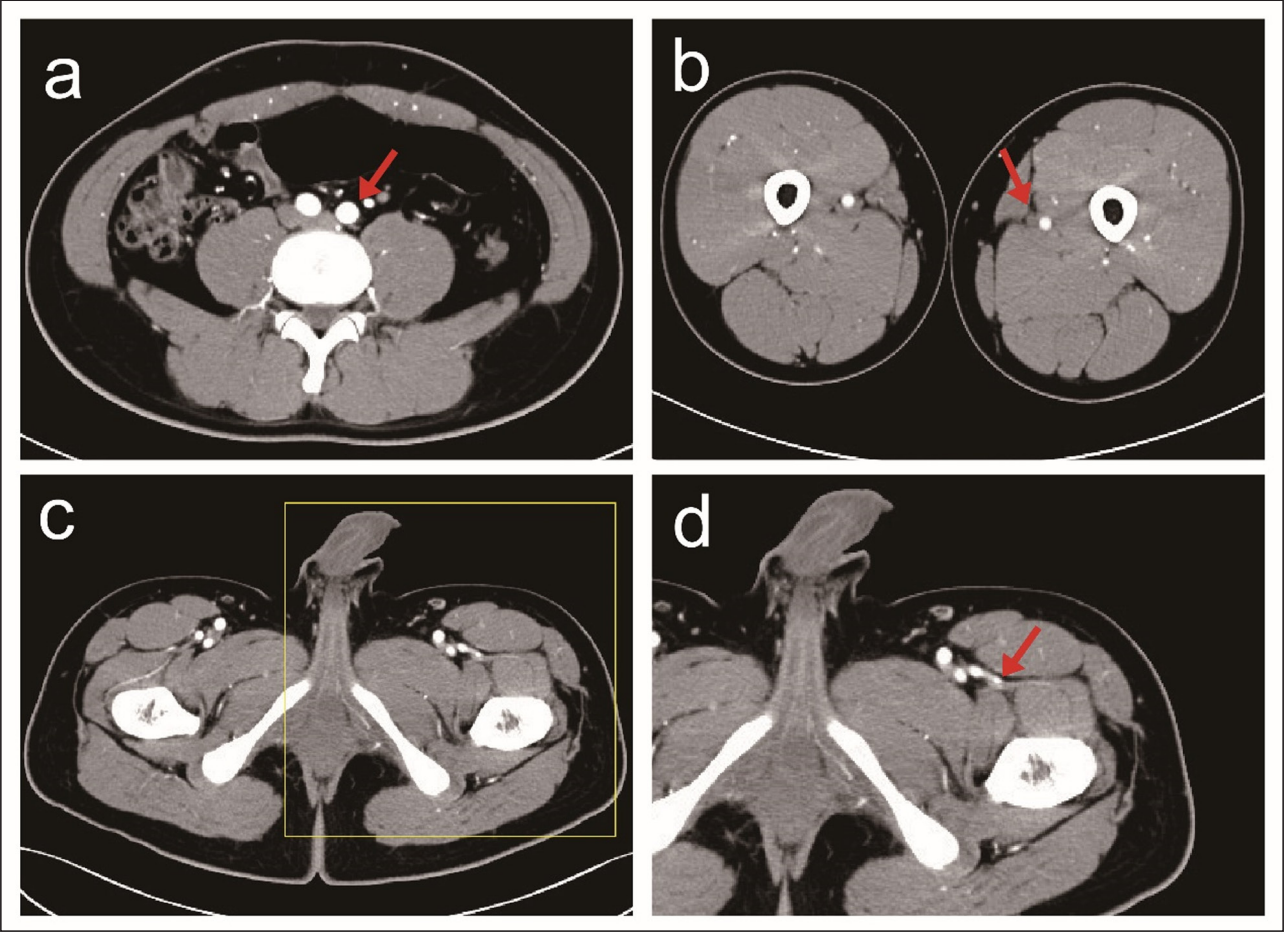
CTA Data Processing. a. The abdominal aorta bifurcation level; b. The mid-level of the left FA; c. The first perforating branch of the descending branch of the left lateral circumflex femoral artery (CFA), penetrated by 1 cm; d. The first perforating branch of the descending branch of the left lateral CFA, with 10 times magnified.
